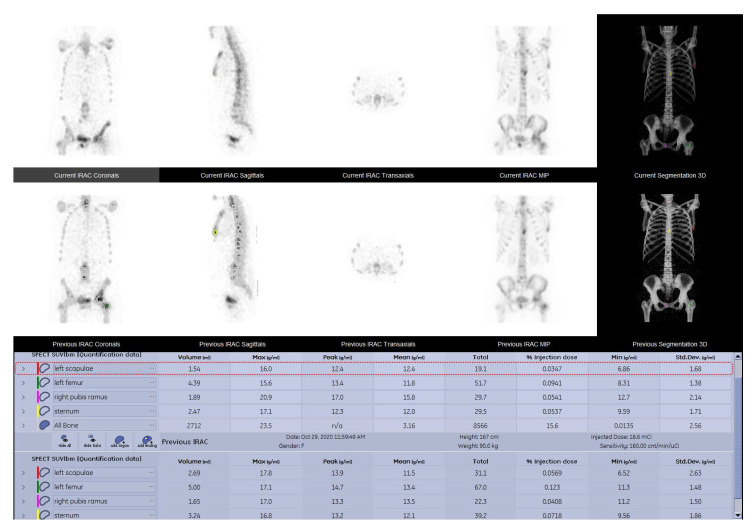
SPECT-CT data quantitative analysis results displayed in comparison between the baseline and the follow up study of a 52-year-old female breast cancer patient with grade two lobular invasive breast carcinoma, presenting multiple metastatic lesions, following chemotherapy and osteoclast inhibitor.
